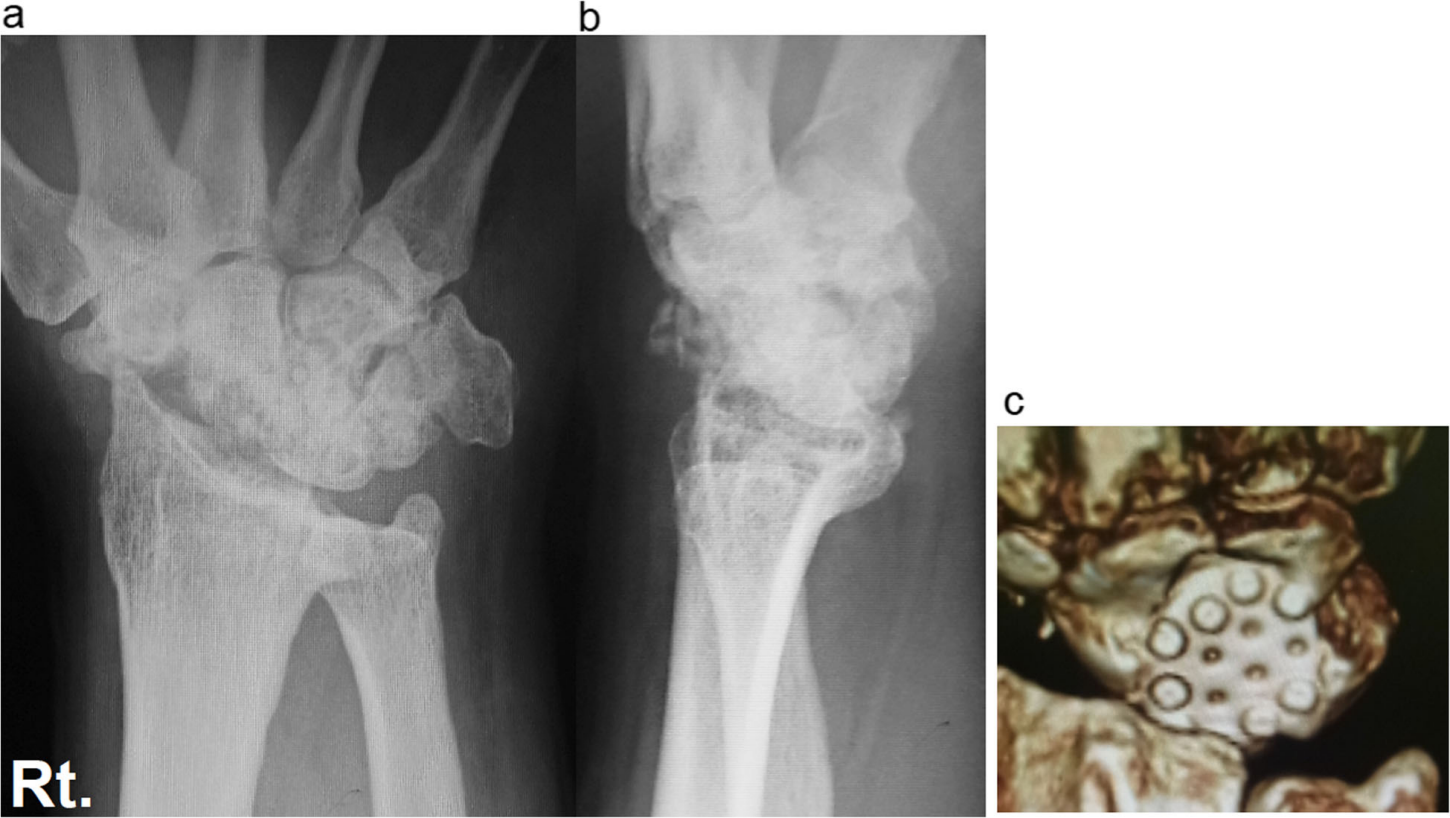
Postoperative X-rays (a, b) and three-dimensional computed tomography scan (c). Fifty-six-year-old male patient at the one-year follow up
